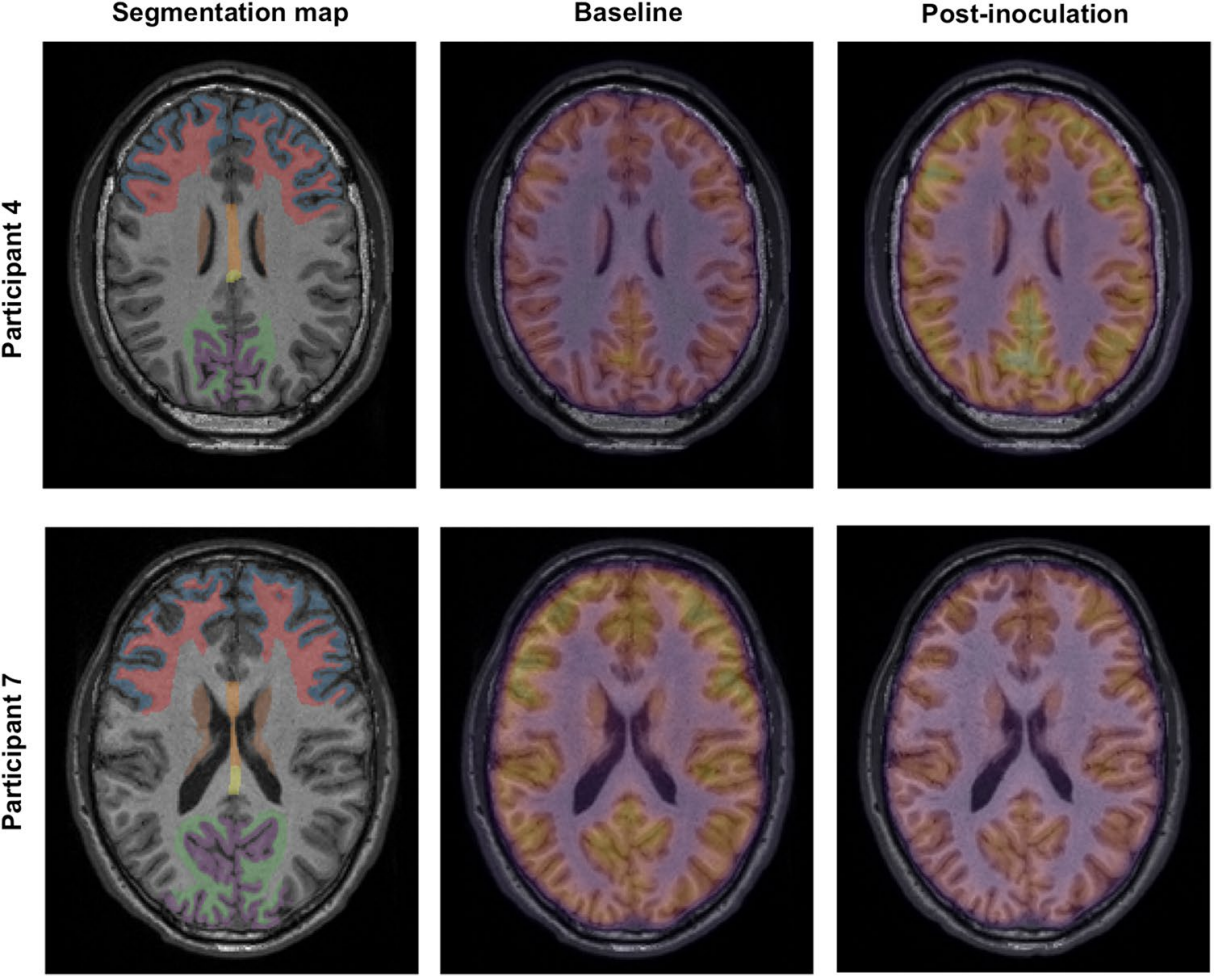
Example posterior subregion segmentations of T1-weighted MRI sequence (left panels), whole brain radiotracer uptake at baseline (middle panels) and post-inoculation (right panels) in Participant 4 (top row) and Participant 7 (bottom row). Segmentation map: blue—anterior grey matter; red—anterior white matter; brown—deep grey matter; yellow/orange—corpus callosum (yellow indicates splenium); purple—posterior grey matter; green—posterior white matter. Participant 4: Increased radiotracer uptake is evident globally on post-inoculation imaging. Participant 7: Decreased radiotracer uptake is evident globally on post-inoculation imaging.
